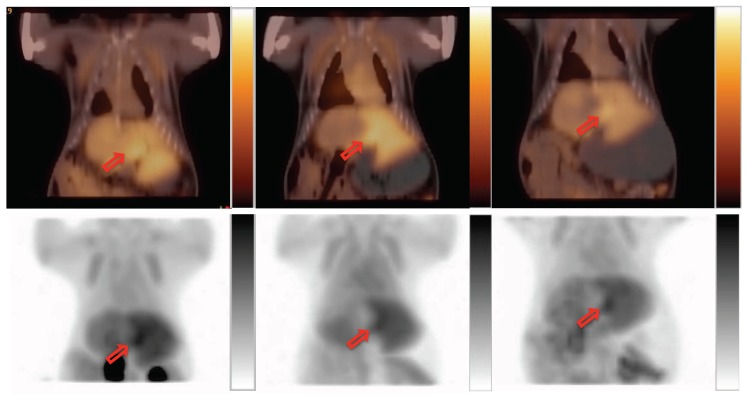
Three-way comparison of [^18^F]CFA uptake in tumor (red arrow) and liver. From the left: Baseline, post UGT inhibitor probenecid application, and after cold CFA application. In each column, the upper panel is PET/CT overlay, while the lower panel is the maximal intensity projection (MIP).
